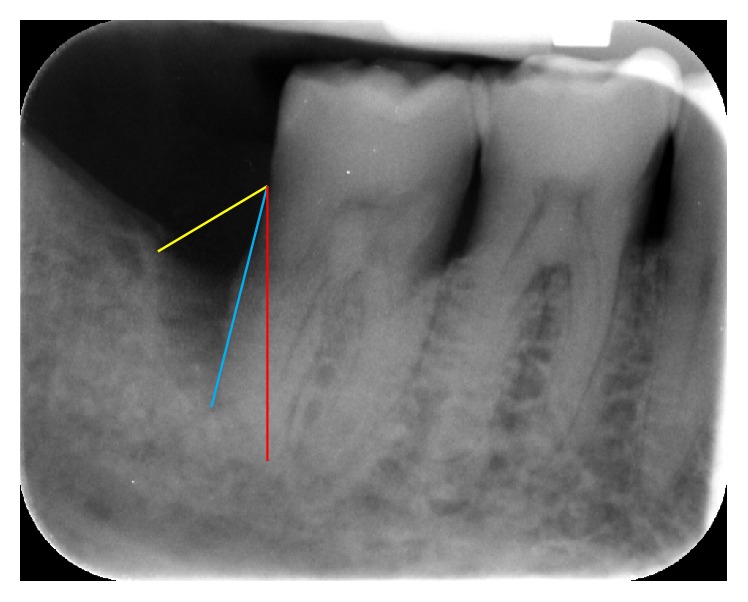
Radiographic measurements to measure changes in relative linear bone height. Root length from CEJ to apex (red line), CEJ to coronal extent of bone height (yellow) on the root surface, and CEJ to most apical extent of bone defect (blue).
